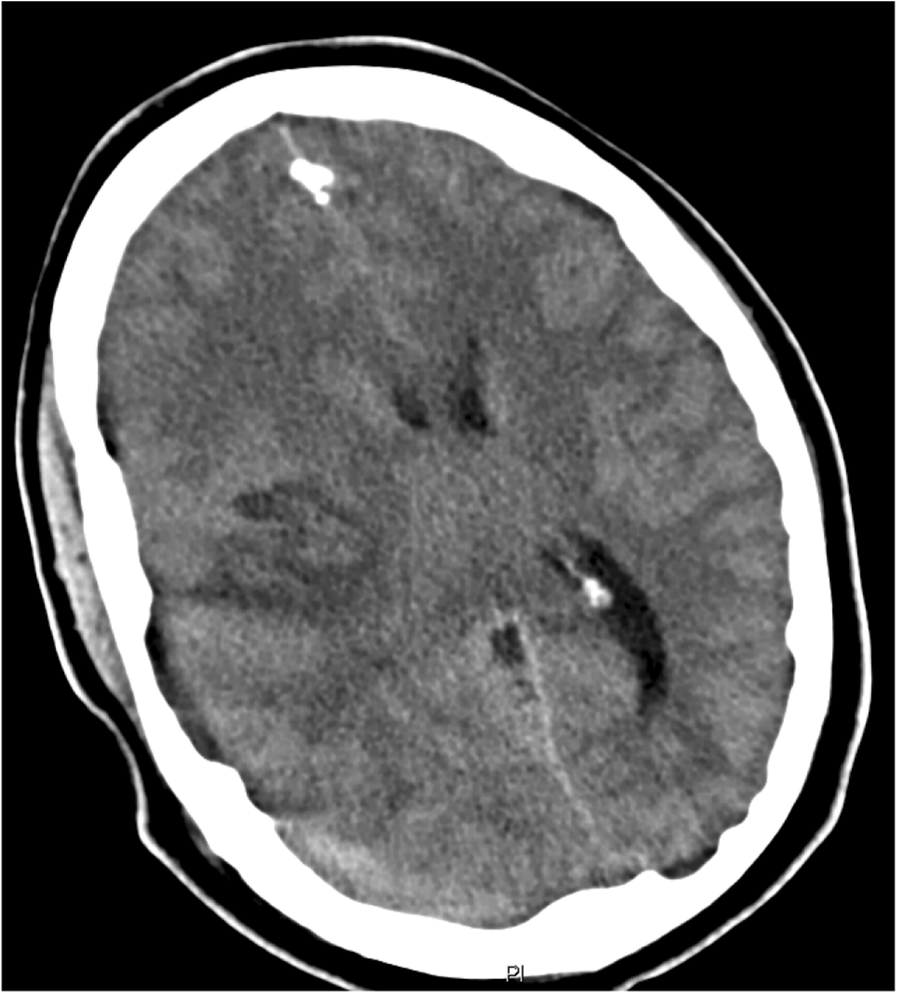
Computed tomography (axial) demonstrating a right-sided lesion of indeterminate nature causing mass effect and significant midline shift
